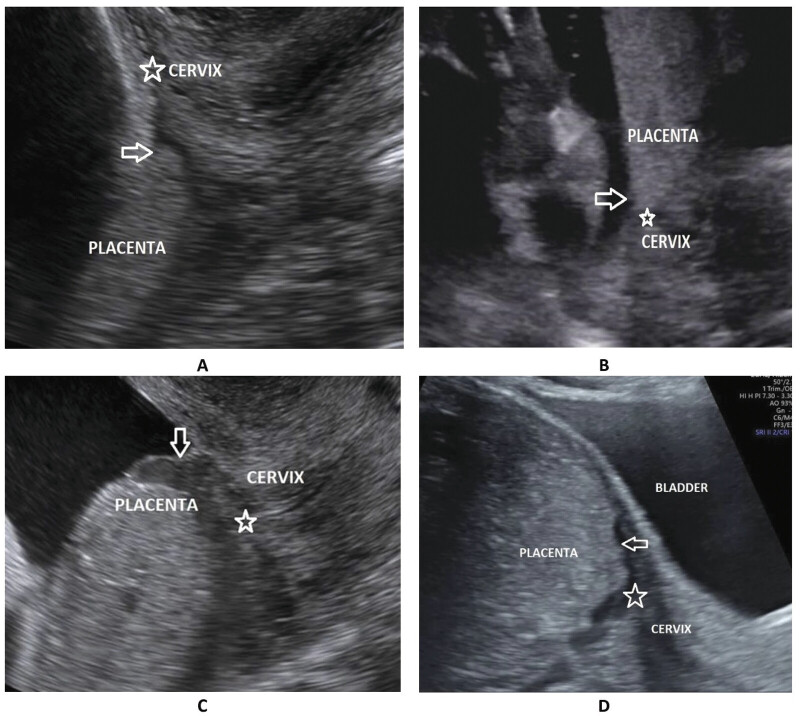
The classification of placenta previa depends on the distance between the lower edge of the placenta (white arrow) and the internal cervical os (white star). This classification on ultrasound includes type I, low-lying placenta (
